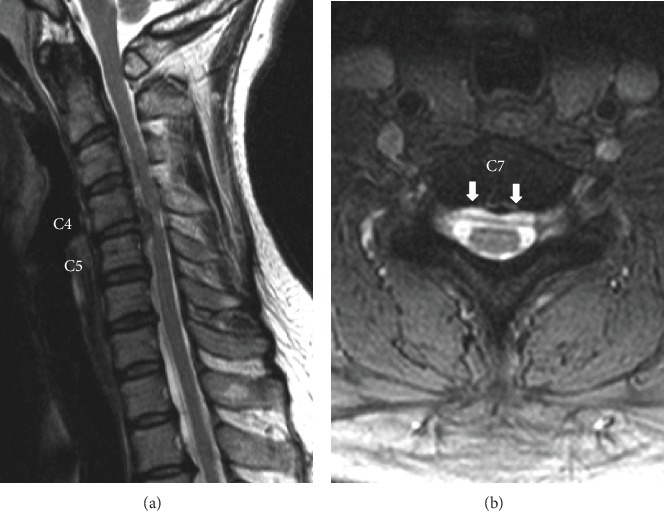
Case 2. Preoperative T2-weighted MRI. (a) Sagittal view: cervical disc herniation compressing the spinal cord was observed at C4-C5. (b) Axial view at the C7 vertebra level: fluid collection was observed on the ventral side of the dura (white arrows).
